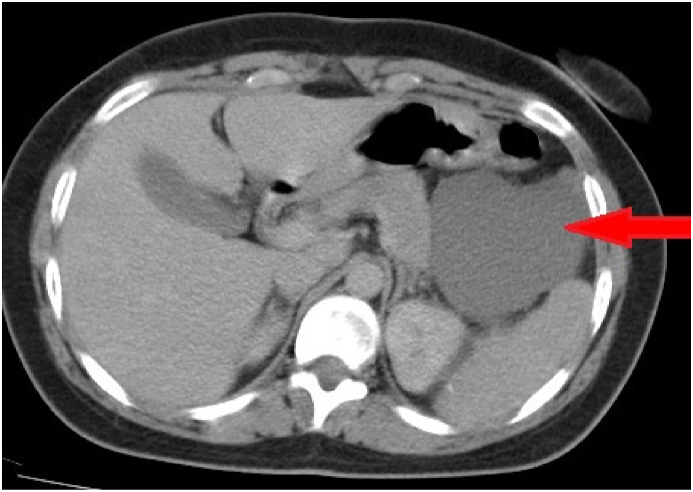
Abdominal CT scan showing a cystic mass in contact with the stomach, the tail of the pancreas, the spleen, and the Antero external cortex of the left kidney.
